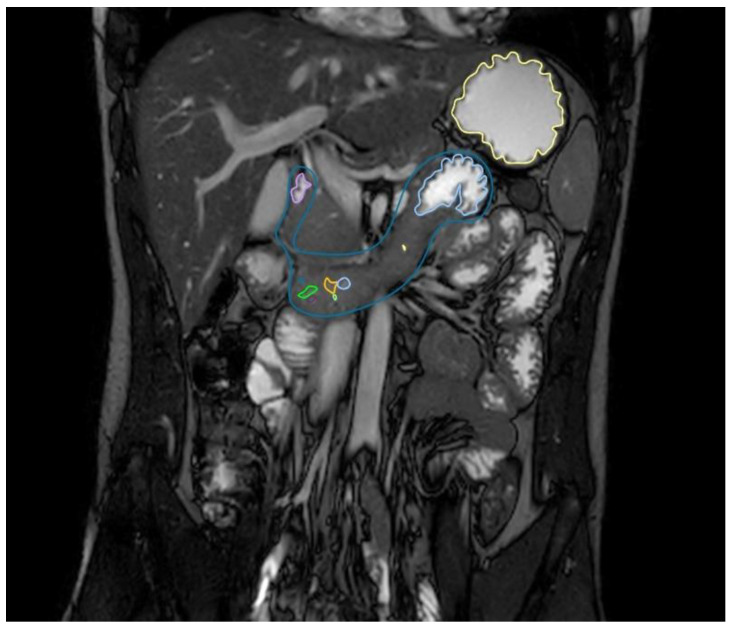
MRI TrueFISP sequence image in the coronal plane; the stomach (yellow outline) and duodenum (dark-blue outline) with the fluid pockets outlined in different colours.
